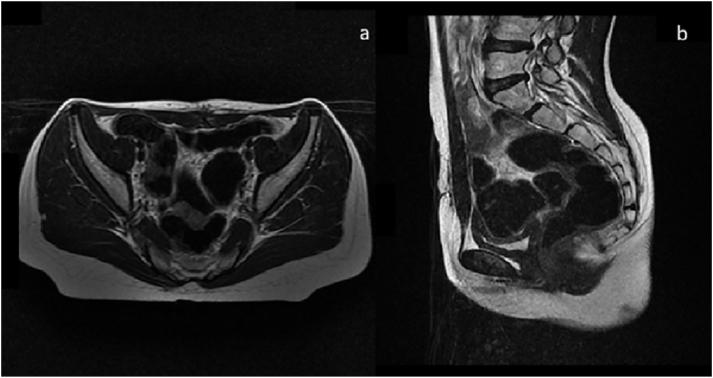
a. transverse and b. Sagittal MRI control after 6 months not showing any recurrence or tumor residue.
